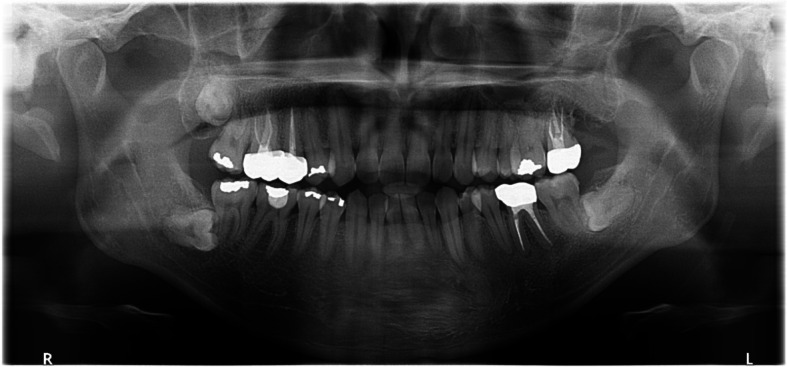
Panoramic radiograph at 1 year and 7 months postoperatively shows no evidence of recurrence
